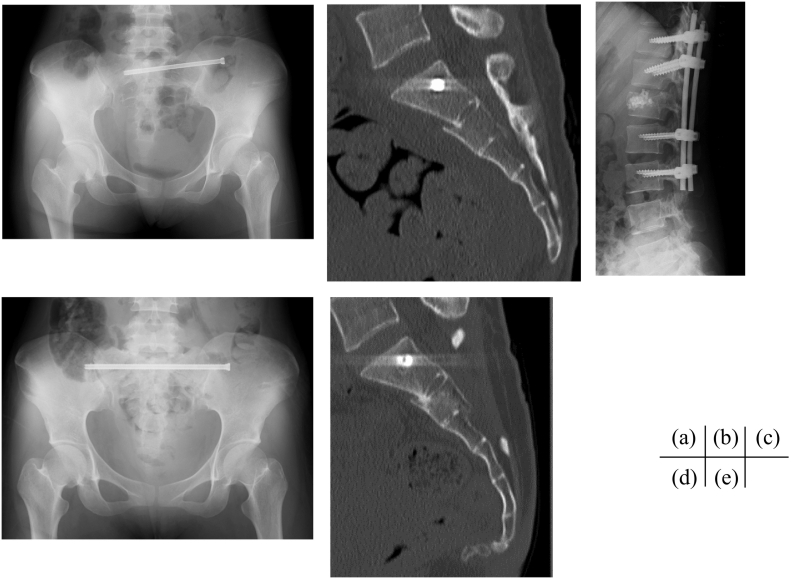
(a) Radiograph of the pelvis and (b) Sagittal computed tomography (CT) image after emergent surgery. The sacral canal at the S2 level was enlarged. (c) Radiograph of the lumbar spine after percutaneous fixation for the L1 burst fracture. (d) Radiograph and (e) CT image of the pelvis at 10 months after the injury.
